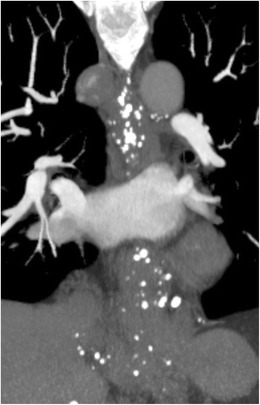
Varices. Maximum intensity projection of a CT angiogram of the chest in soft tissue window shows numerous calcifications along the oesophageal wall and portal collateral vessels in this patient with extensive uphill oesophageal varices
